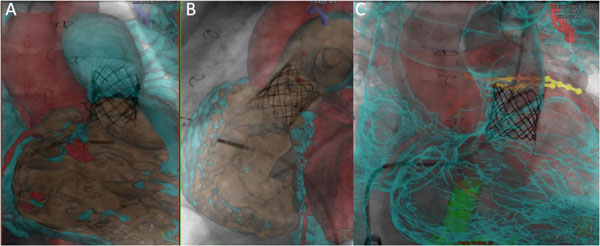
A) Anterior-posterior, and B) lateral biplane X-ray magnetic resonance fusion with two surfaces loaded. The right side of the heart is carved open to visualize the valve placement in the pulmonary artery. C) Contour rendered overlay of the right side of the heart in the cranial projection showing the spatial relation between the valve and the left coronary arteries which were marked with 3D lines to enhance visualization.
